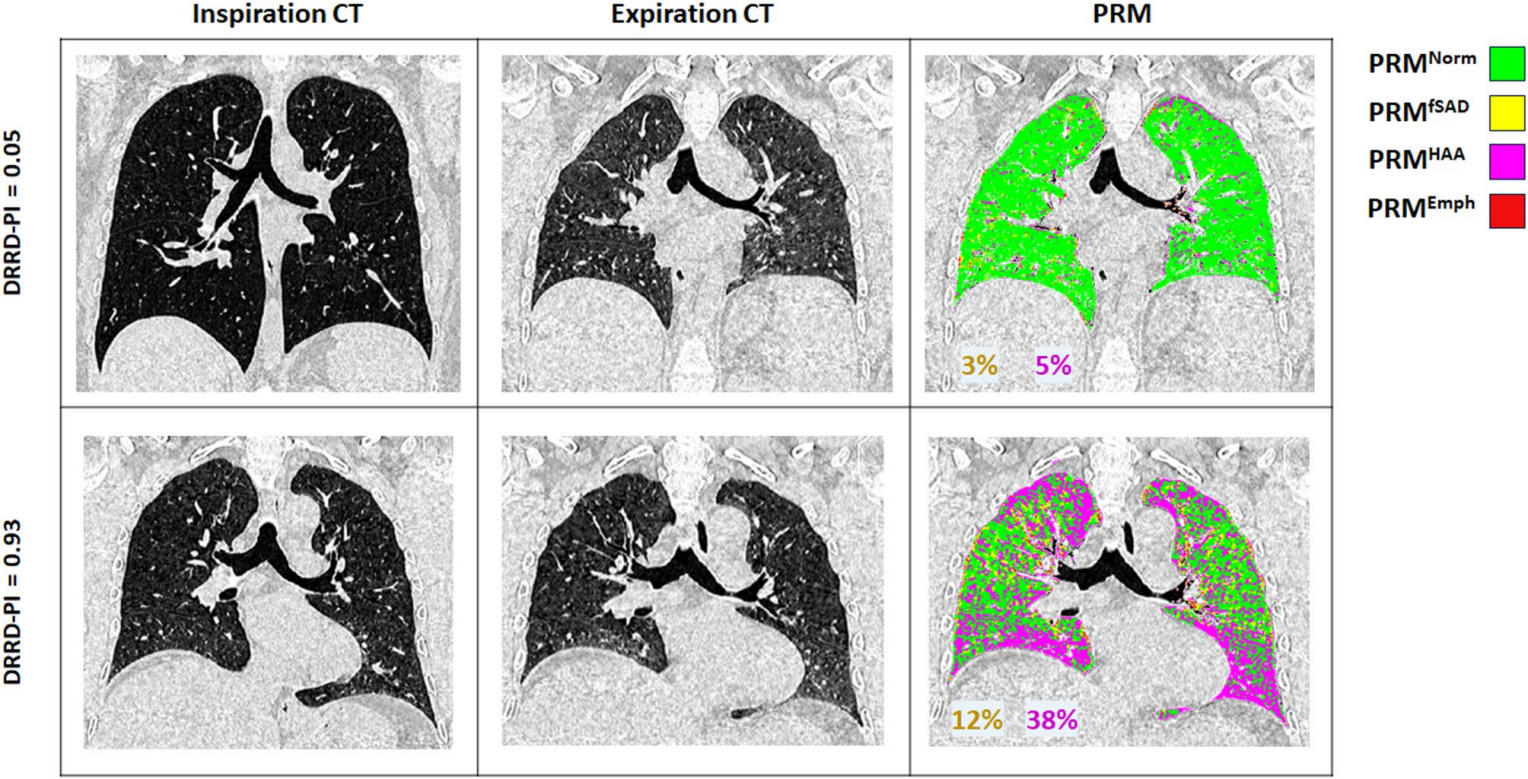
Representative HRCT images from FDSV subjects. Inspiratory (left panels), expiratory (middle panels), and PRM (right panels) images from FDSV subjects with a DRCB-PI < 0.5 (top row) and a DRCB-PI > 0.5 (bottom row). PRM images are color coded to identify lung tissue as normal (green), functional small airways disease (yellow), high attenuation area (pink), and emphysema (red). Percentages of PRM^fSAD^ (yellow text) and PRM^HAA^ (red text) are provided for additional context
